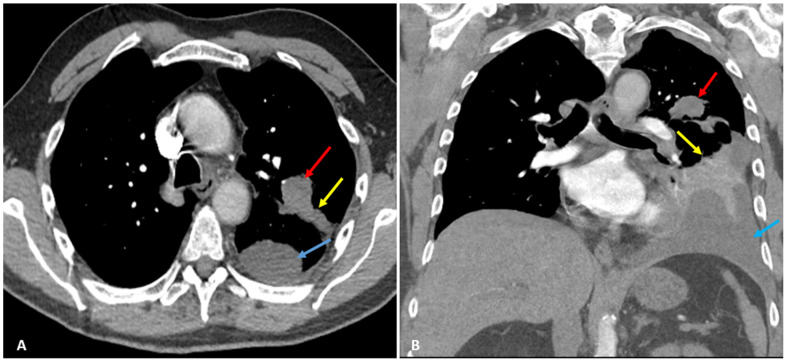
Axial (A) and coronal (B) CT scan with IV contrast shows left upper lobe peri-bronchial mass (red arrows) measuring approximately 3 × 2 cm in diameter. Distal obstructive atelectasis (yellow arrows) and left pleural effusion (blue arrows) are observed as well.
